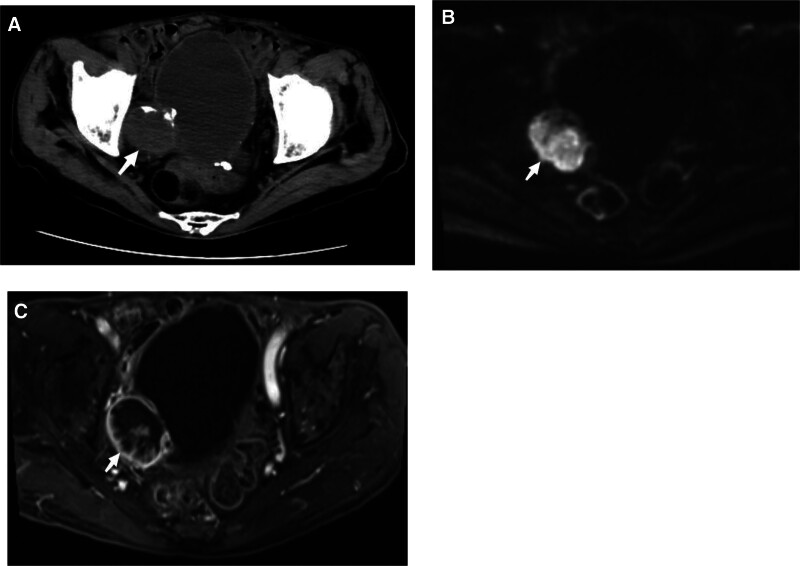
Radiographic features of the patient. (A) Pelvic CT: A mass (arrow) of approximately 34.9 × 25.7 × 35.2 mm was found on the right side of the bladder, and the mass was exophytic and partially calcified inside. (B) Pelvic MRI: the mass (arrow) had a high diffusion-weighted signal. (C) Pelvic MRI: the mass (arrow) was significantly enhanced on the dynamic enhanced image.
